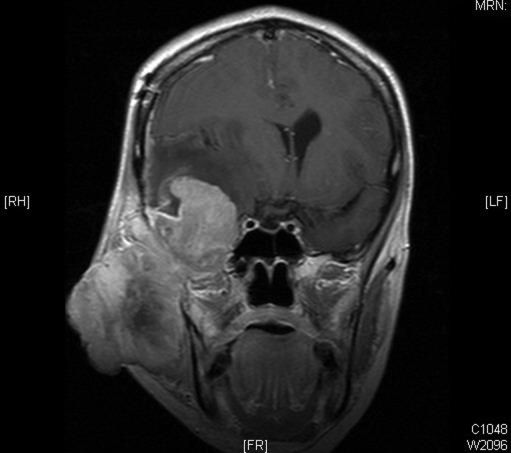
Coronal T1 post contrast MR image showing the tumour extension intracranially into the right temporal lobe causing compression of the right lateral ventricle and midline shift to the left.
